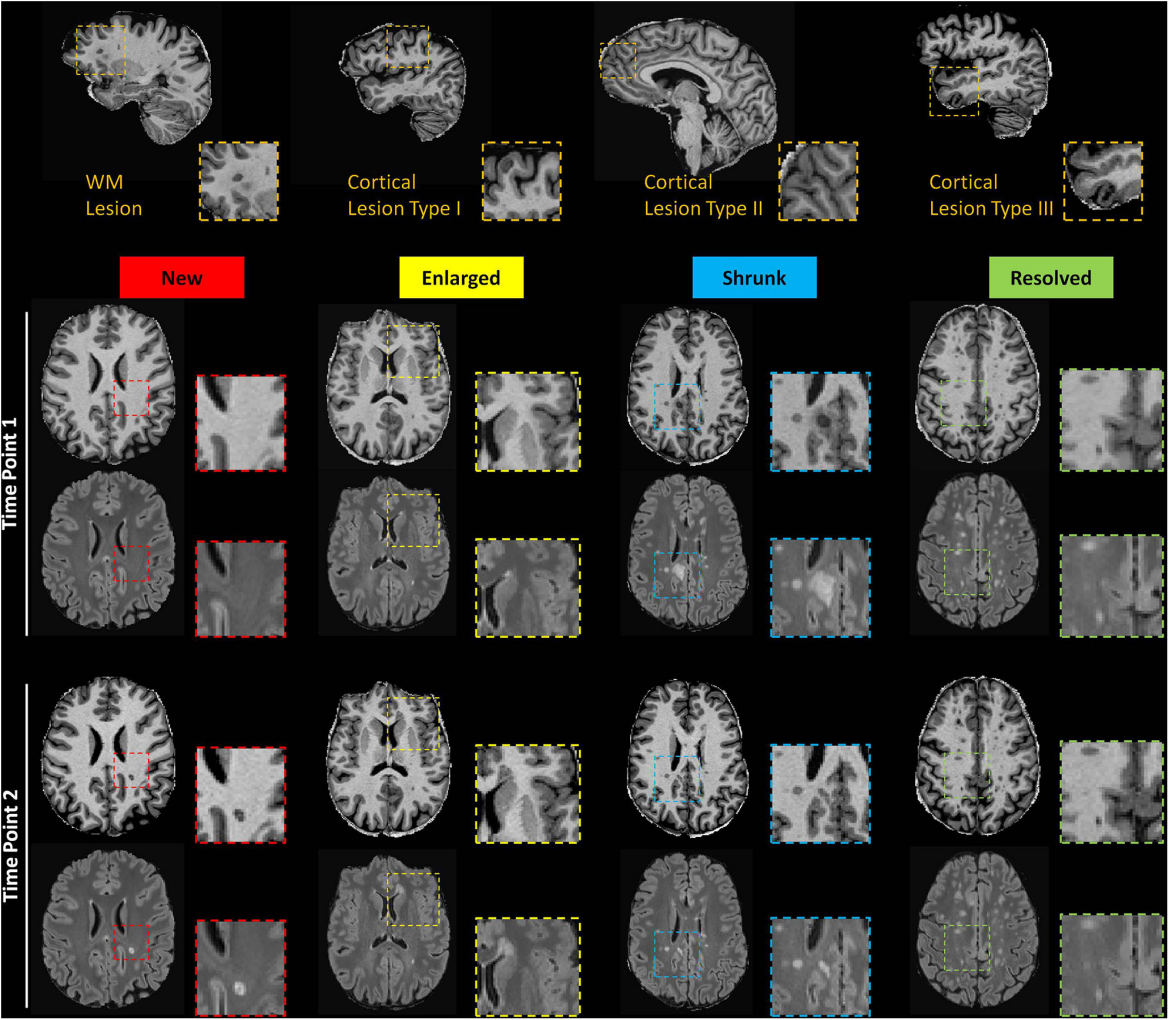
Top row: Exemplary sagittal view in one patient showing WML and CL type 1, 2, and 3. Bottom rows: Axial slices of MP2RAGE and 3D FLAIR images showing exemplary new, enlarged, shrunken and resolved WML as automatically detected.
